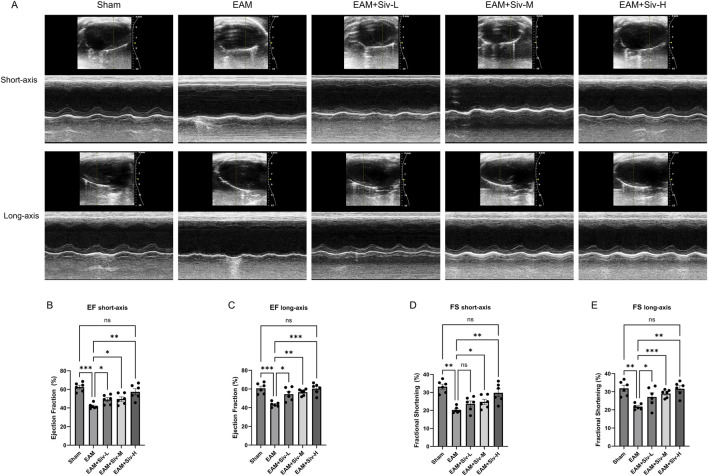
(A) Representative short-axis and long-axis M-mode echocardiographic images at day 21 post-immunization. (B–E) Quantification of left ventricular ejection fraction (LVEF) and fractional shortening (LVFS) from short-axis (B,D) and long-axis (C,E) views. Data are mean ± SEM (n = 6). (Sham vs. EAM and EAM vs. EAM + Siv). p* < 0.05, *p* < 0.01, p* < 0.001; ns, not significant (one-way ANOVA with Bonferroni’s post-hoc test).
